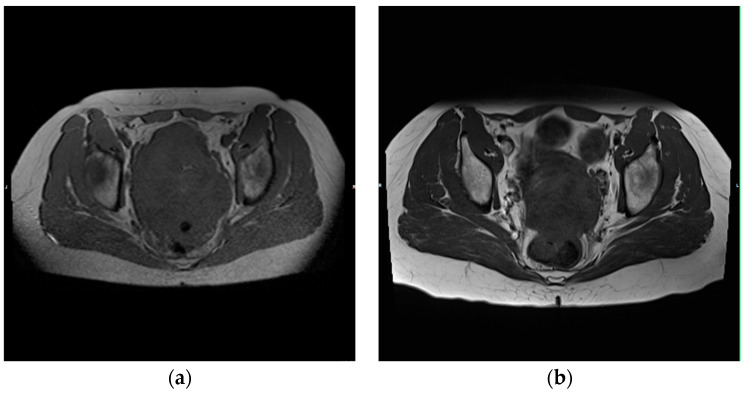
A 47-year-old patient diagnosed with ovarian carcinoma underwent eight TACP procedures. Following the completion of the treatment cycle, the measurements demonstrated a reduction of 34% in the longest diameter. Consequently, the patient exhibited a partial response. (a) Transverse T1-weighted image of the pelvis that depicts the tumor prior to the initiation of therapy; (b) Transverse T1-weighted image of the pelvis that shows the tumor following the completion of eight TACP procedures, which resulted in a significant reduction in the longest diameter.
